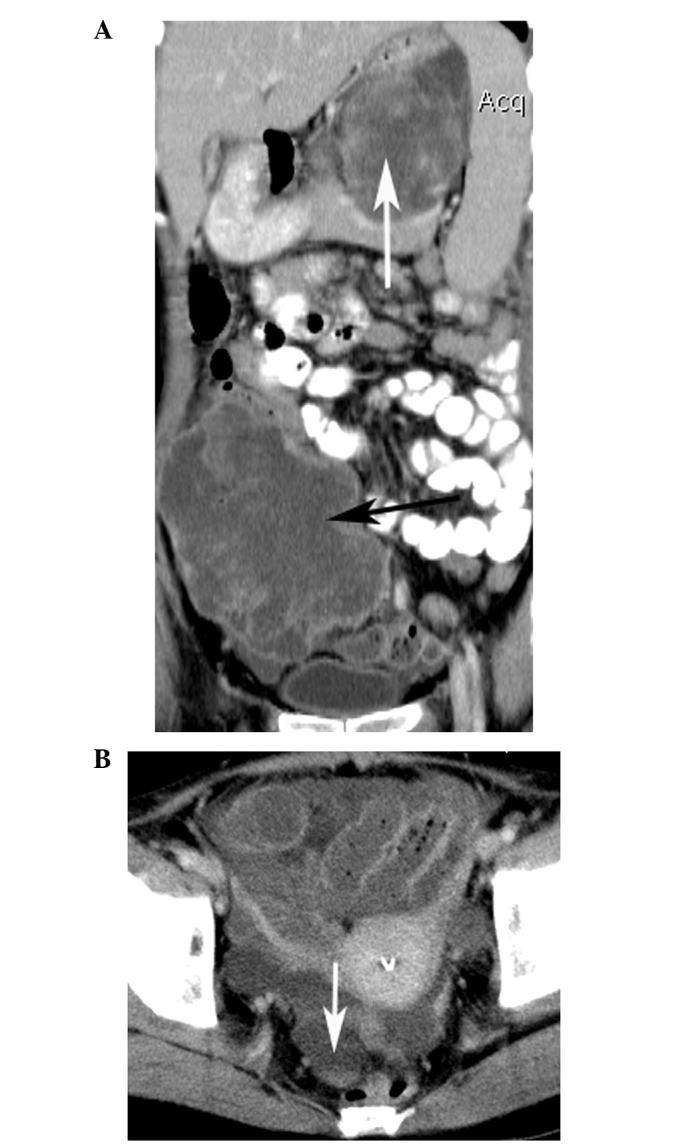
A 42-year-old female with a high-grade extragastrointestinal stromal tumor in the mesentery. (A) Coronal contrast-enhanced computed tomography (CT) image showing a primary mass in the ileocecal region (black arrow) and a metastasis in the adrenal gland (white arrow) with heterogeneous enhancement. (B) Axial CT image showing pelvic effusion (arrow).
